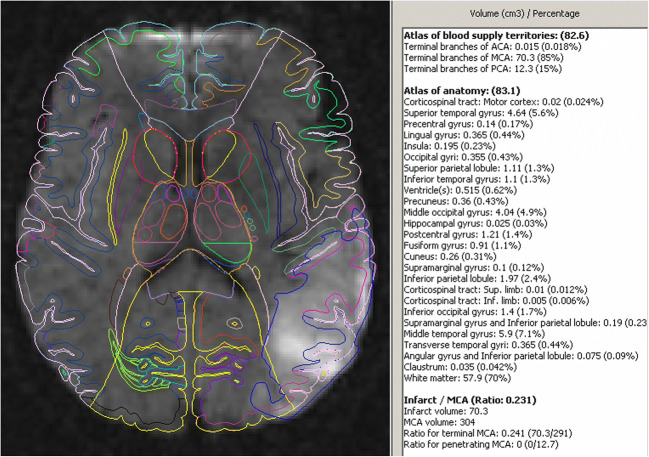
Atlas-assisted treatment decision making in ischemic stroke: left) a DWI axial image with a delineated infarct (in blue) and the individualized anatomic and blood supply territories atlases in contour representation superimposed on the image; right) atlas-assisted analysis with a contribution of the blood supply territories (top) and anatomic structures (middle) to the infarct in terms of name, volume in cm^3^ and percentage as well as the assessment of the ratio of the infarct to the whole middle cerebral artery (MCA) territory including that for the terminal and penetrating branches (bottom)
